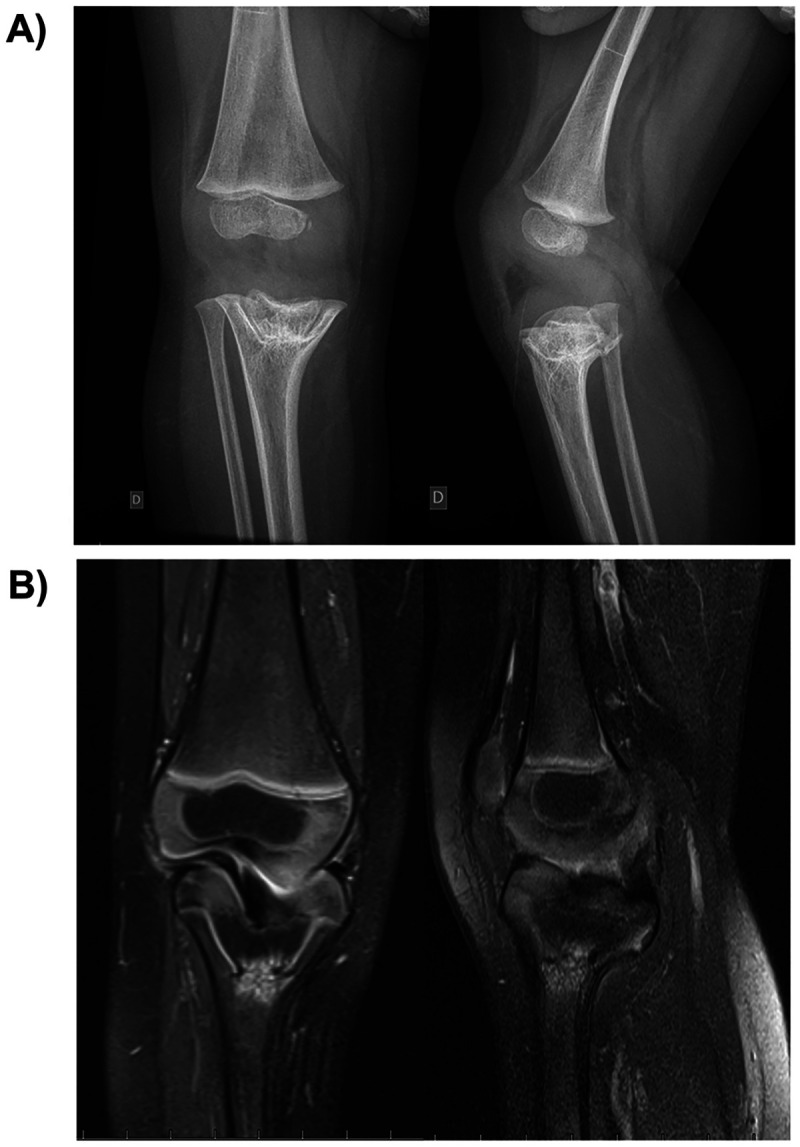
(A) primary anteroposterior and lateral radiographs of the right knee showing severe damage to the proximal tibial physis, with a depression of the epiphysis into the metaphysis, suggestive of a central physeal bar involving approximately 33% of the physeal surface. (B) MRI of the right knee showing a markedly concave deformity of the proximal tibial epiphysis, with a central fossa and cupping, associated with significant remodelling of the adjacent medial femoral condyle.
